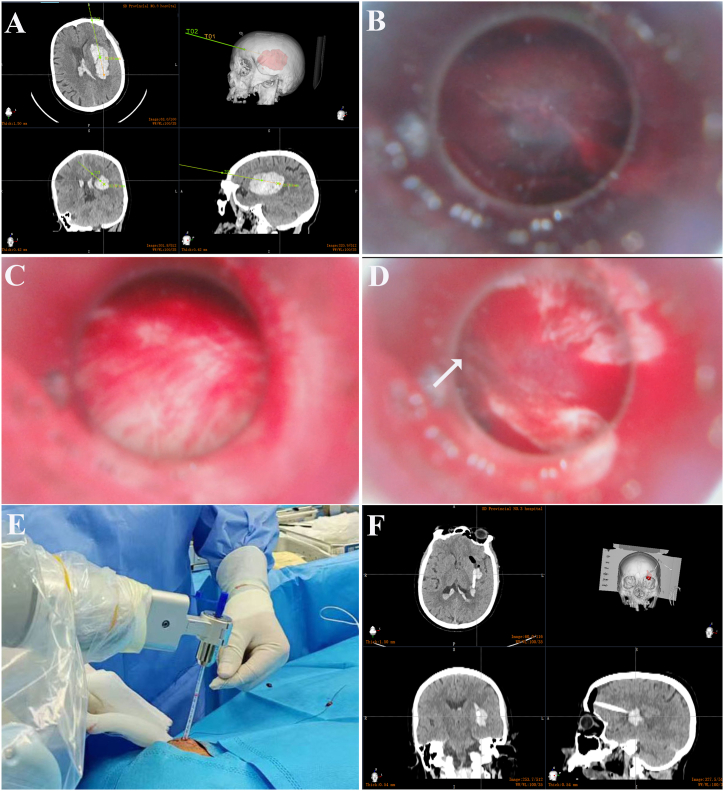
The RAVMIA technique for case 2. The surgical trajectory along the longitudinal axis of the hematoma was established by the robot (A). Endoscopy revealed a solid-like hematoma in the bottom layer (B), which was completely evacuated through aspiration (C). The same procedure was repeated in the top layer. The entire trajectory endoscopy revealed a residual hematoma at a depth of 77 mm and oriented at 9 o'clock (D). Targeted aspiration was performed using a biopsy needle, followed by the placement of a drainage tube (E). A postoperative CT scan confirmed the successful evacuation of the hematoma (F). (arrow: residual hematoma).
